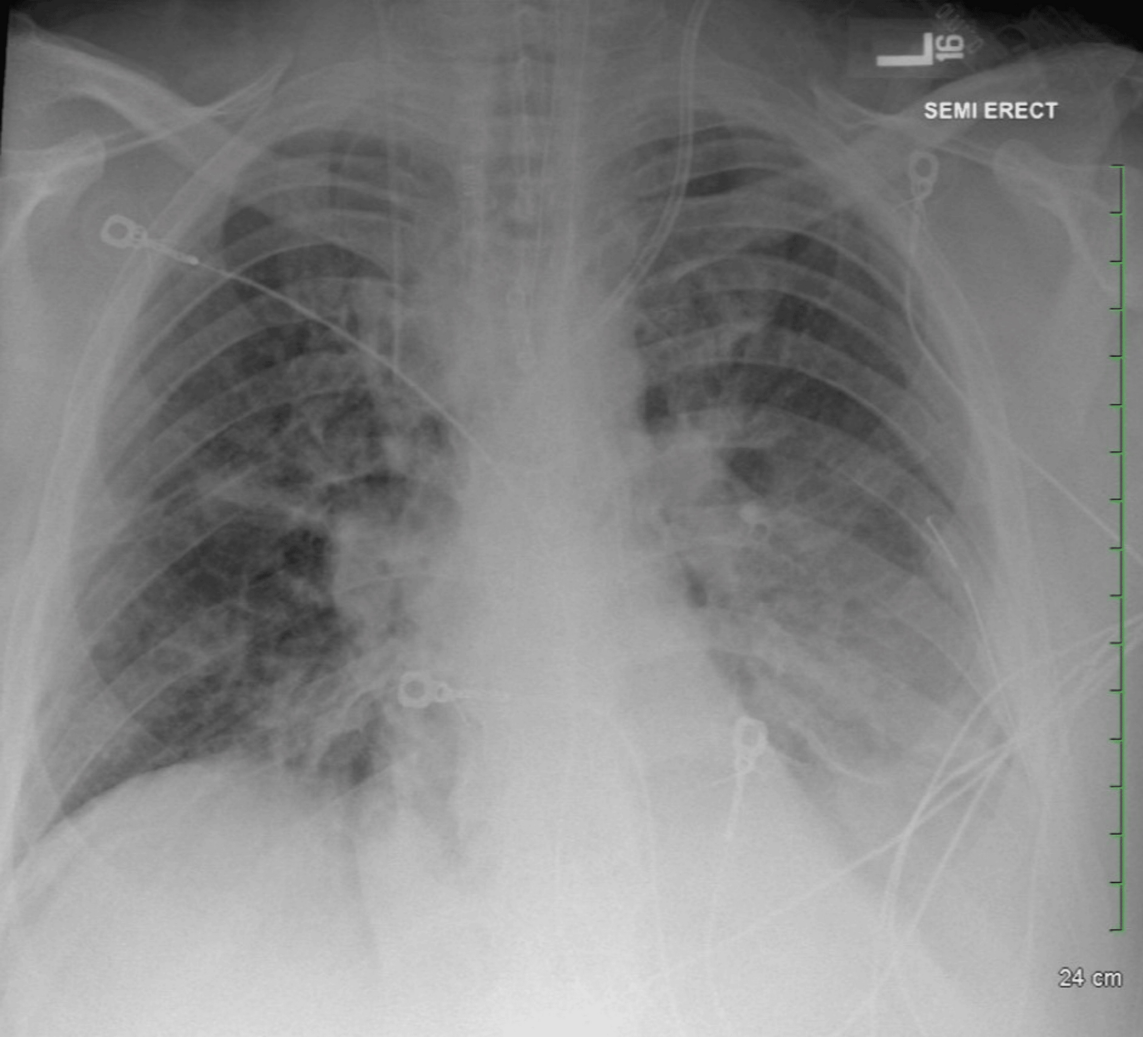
Chest X-ray following pigtail removal and surgical chest tube insertion
